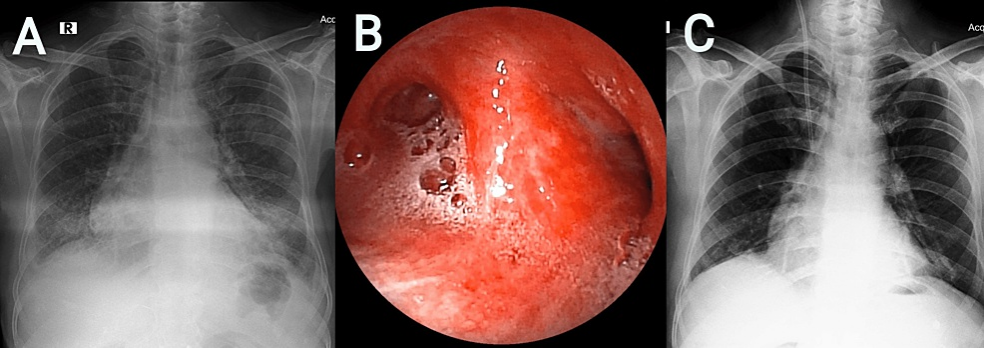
A) Chest radiograph revealed bilateral diffuse infiltrates. B) Flexible bronchoscopy revealed frothy secretions. C) Resolution of pulmonary edema in chest radiograph.
